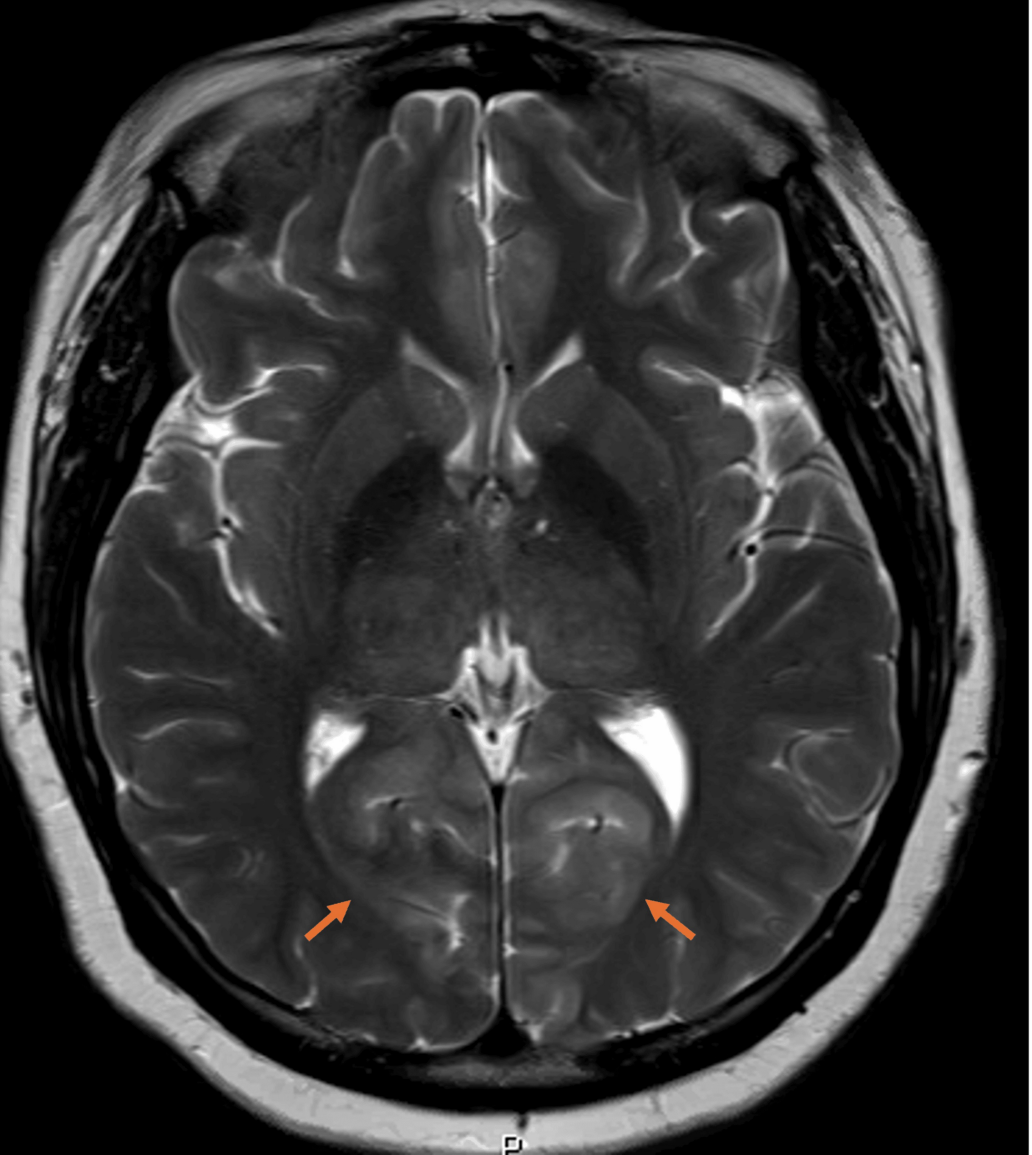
MRI of the brain T2 sequence shows corresponding occipital high signal intensity (arrows)
